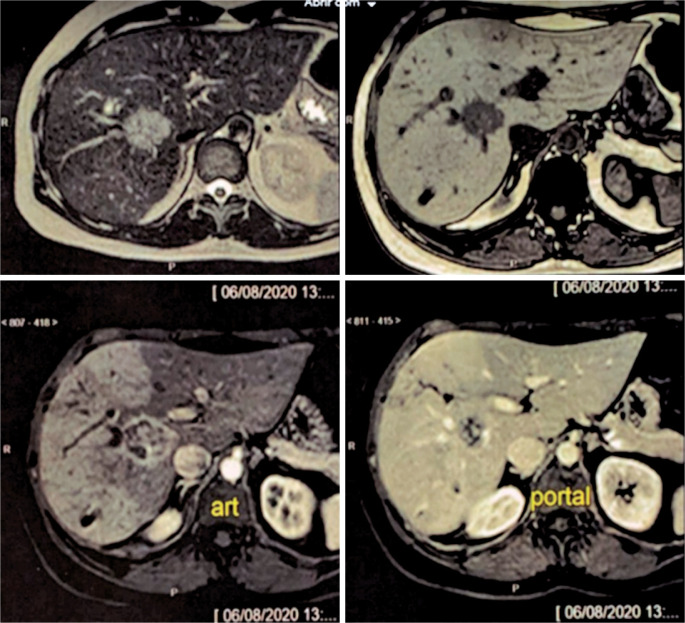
Magnetic resonance imaging after embolization showing occlusion of the right hepatic vein and the right branch of the portal vein
